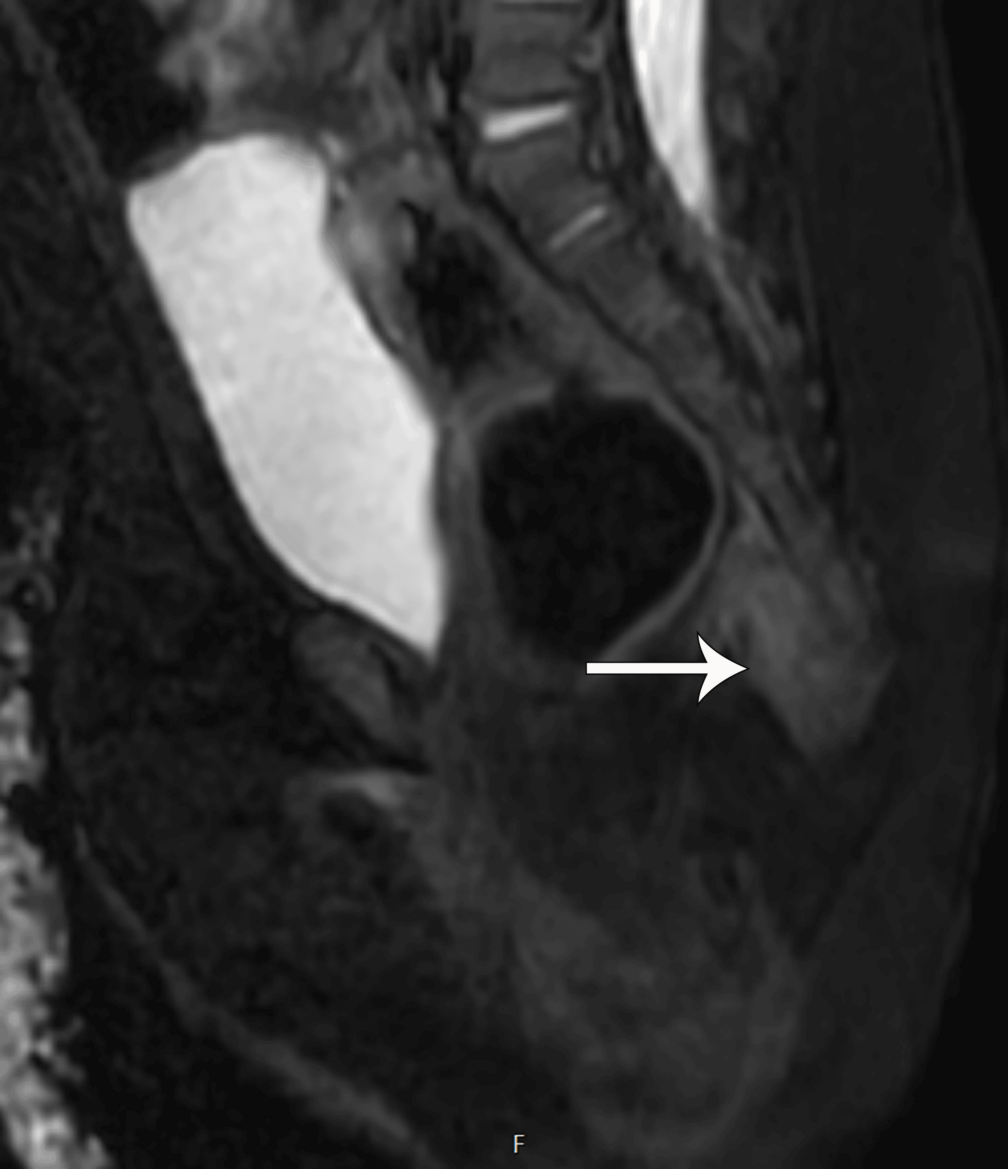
The lesion on T2-weighted STIR sagittal MRI image showing homogeneous mild hyperintensity (white arrow). No cystic components or necrosis. No bone marrow edema pattern of the coccyx
